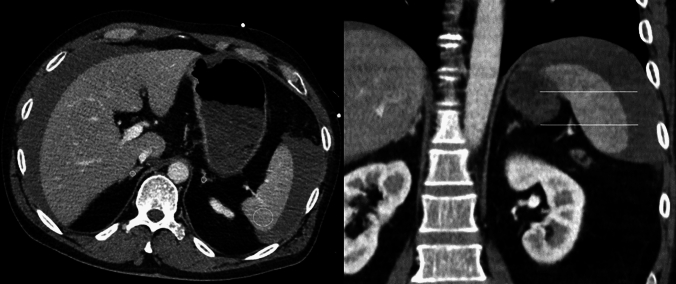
Representative patient of our patient sample with an axial and coronal CT image in portal venous contrast phase. The region of interest placement is drawn within the boundaries of the adrenal glands and the spleen to measure the radiodensities. Free fluid surrounding the liver and spleen can also be appreciated
